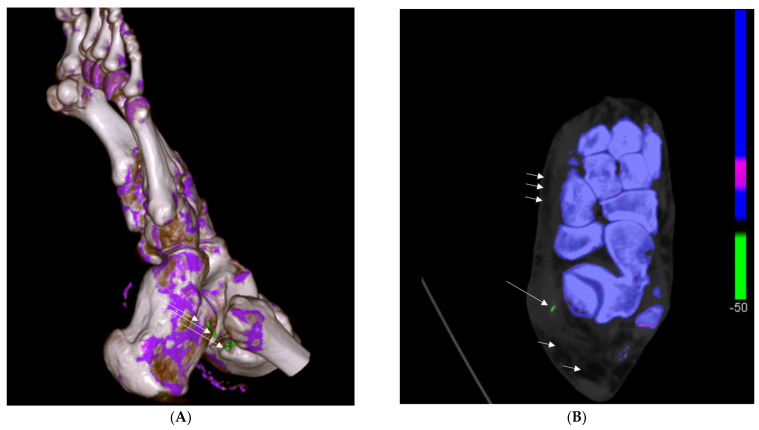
Dual-energy computed tomography (DECT) images of the same patient with extra-articular gout deposits at the peroneal tendon. (A): A 3D DECT reconstruction showing typical green-coded monosodium urate (MSU) deposits (long arrows) along the peroneal tendon. (B): Axial DECT scan of the ankle joint highlighting green-coded MSU deposits (long arrow) and areas of subcutaneous lymphedema (short arrows).
